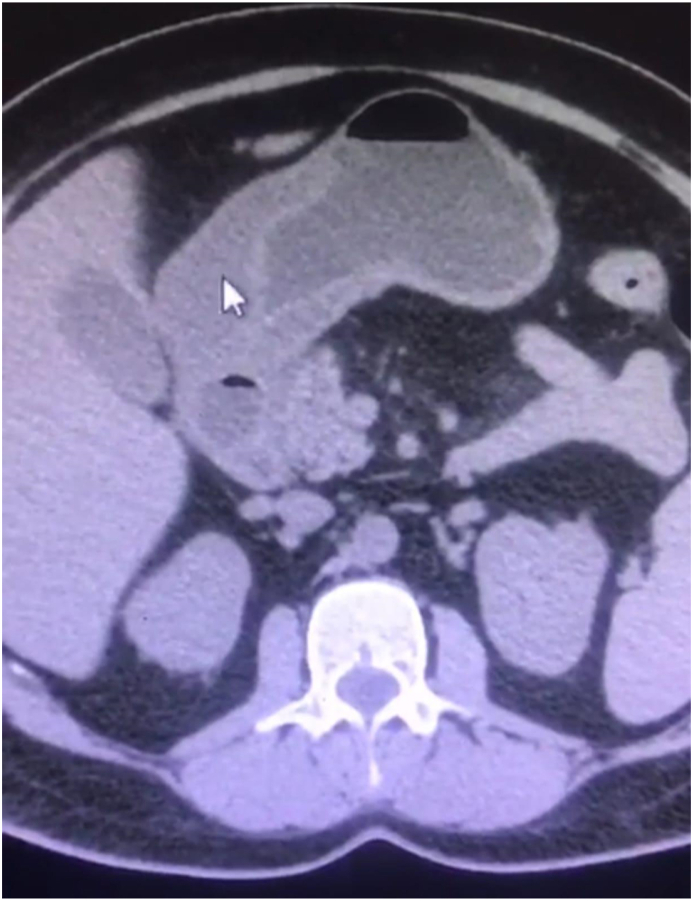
Abdominal CT scan without contrast showing wall thickening involving the pyloric canal and first part of duodenum (arrow).
